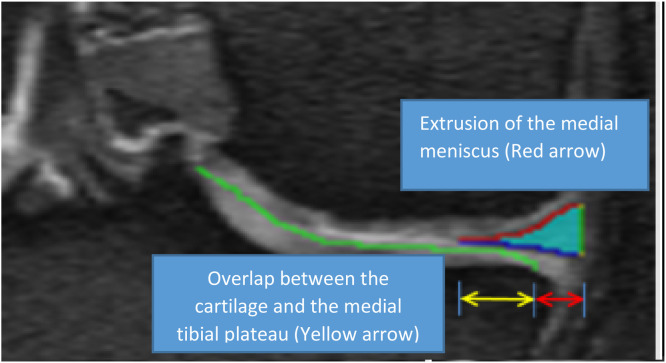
Coronal reconstruction of DESS MRI slice showing the extrusion of the medial meniscus (red arrow), indicating outward displacement of the meniscus beyond the tibial margin.
